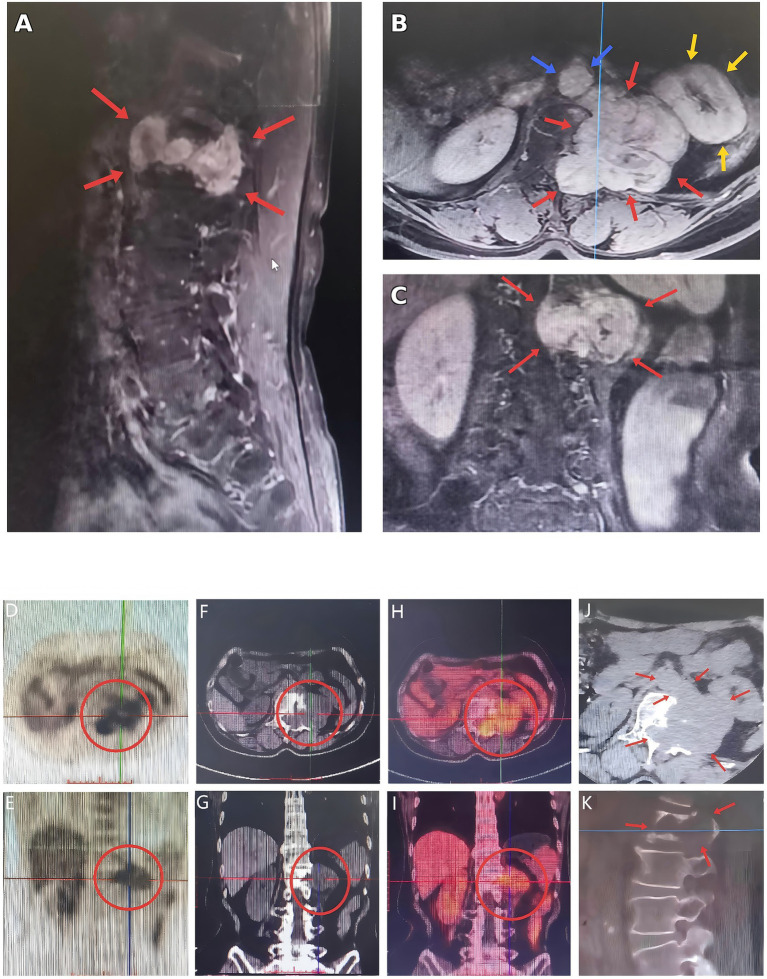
Preoperative contrast-enhanced MRI and [^18^F]FDG PET/CT with CT characterization of a giant dumbbell-shaped schwannoma at the left L1 level. (A) Sagittal contrast-enhanced MRI demonstrates a large, heterogeneously enhancing mass centered at the left L1 pedicle/posterior element region with intraspinal extension and spinal canal compromise. (B) Axial MRI depicts the paravertebral–foraminal–intraspinal components of the lesion and its relationship to adjacent retroperitoneal structures. (C) Coronal MRI further delineates the extraforaminal/paravertebral extent of the tumor. Red arrows indicate the schwannoma; blue arrows indicate the abdominal aorta; yellow arrows indicate the left kidney. (D,E) Maximum-intensity projection (MIP) and multiplanar PET views demonstrate focal [^18^F]FDG uptake corresponding to the lesion (red circles). (F,G) Non-contrast CT reconstructions show a destructive mass centered at the left L1 posterior elements/vertebral body with osteolytic erosion and extension toward the spinal canal and paravertebral space (red circles). (H,I) Fused PET/CT images confirm spatial concordance between increased [^18^F]FDG activity and the CT-defined mass (red circles). (J,K) Additional diagnostic CT images highlight osseous involvement and mass effect at the left L1 level (red arrows). Overall, the imaging findings are consistent with a dumbbell-shaped lesion spanning the paravertebral, foraminal, and intraspinal compartments.
